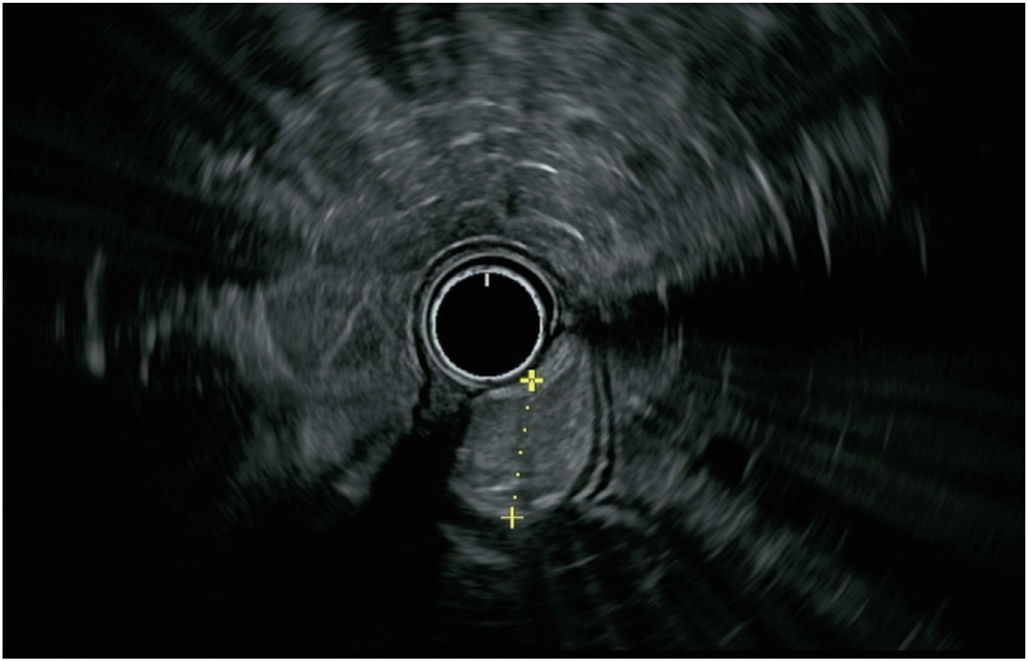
Radial EUS demonstrated a hyperechoic mass with well-defined endosonographic borders arising from the submucosal layer, consistent with lipoma.
